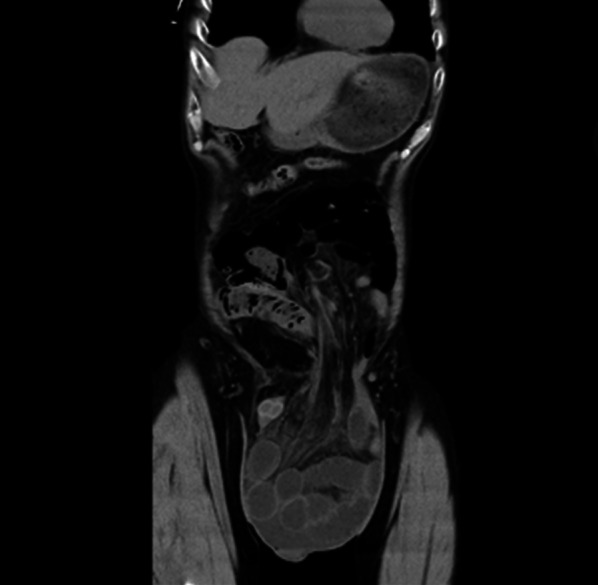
Computed tomography finding of a giant inguinoscrotal hernia
